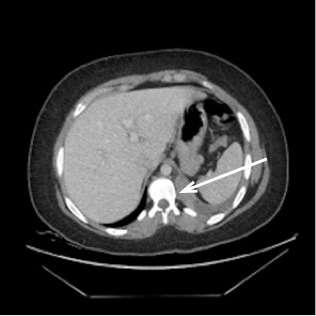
Computed tomography image of thorax with pulmonary sequestration present at left base.
